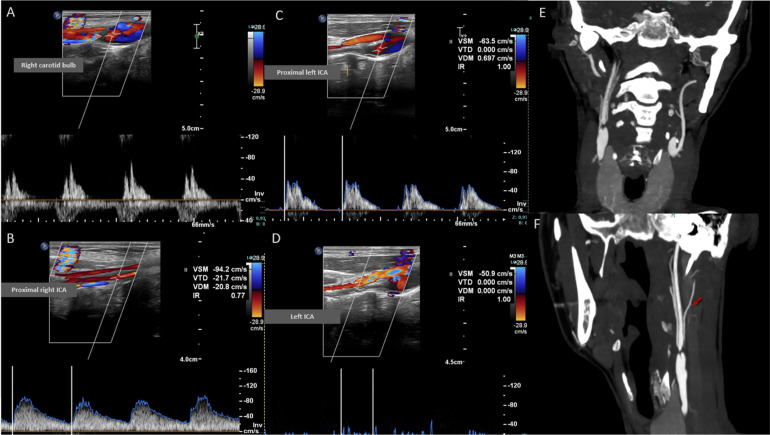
Cervical neurosonology and CTA showing bilateral ICA occlusion with asymmetric collateral reperfusion. (A) Right carotid bulb: high-resistance waveform with absent diastolic flow, indicating distal ICA outflow obstruction. (B) Proximal right ICA: dampened but preserved antegrade flow, consistent with collateral-dependent patency. (C–D) Left ICA: markedly reduced systolic velocities with absent diastolic flow, showing near-occlusion and functional hemodynamic collapse. (E–F) Cervical CTA: bilateral cervical ICA occlusion; right ICA distally reperfused via a collateral artery, highly suggestive of a persistent embryologic carotid–vertebrobasilar anastomosis, while the left ICA remains critically narrowed without effective reconstitution.
